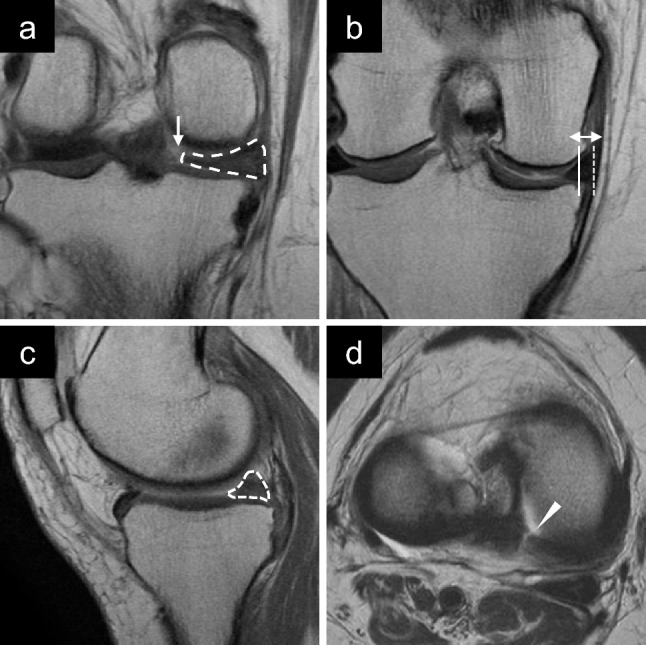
Preoperative magnetic resonance images of type 2 medial meniscus (MM) posterior root tears in the right knee. a Coronal view of the MM posterior portion showing a cleft sign (arrow) and a giraffe neck sign (dashed area). b Coronal view of the middle portion of the MM showing a medial extrusion (double arrow). c Sagittal view showing a ghost sign (dotted area). d Axial view shows a radial tear of the MM posterior root (arrowhead)
